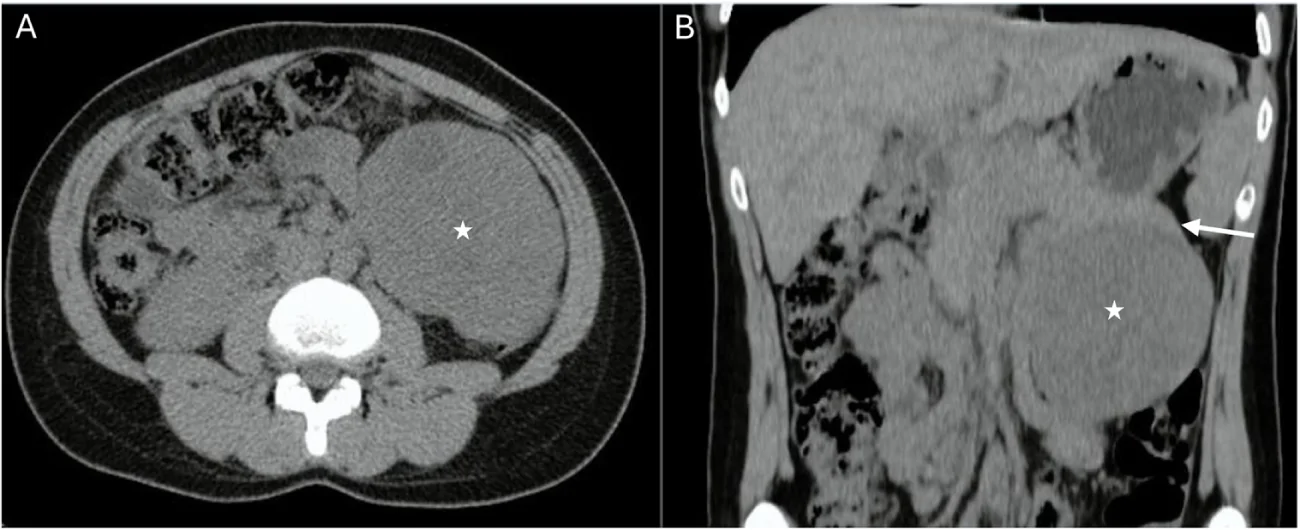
CT scan of the abdomen – axial cut (A) and coronal cut (B) – showing a well-circumscribed mass (identified by a star) without a clear cleavage plane with the pancreatic tail (identified by an arrow).
